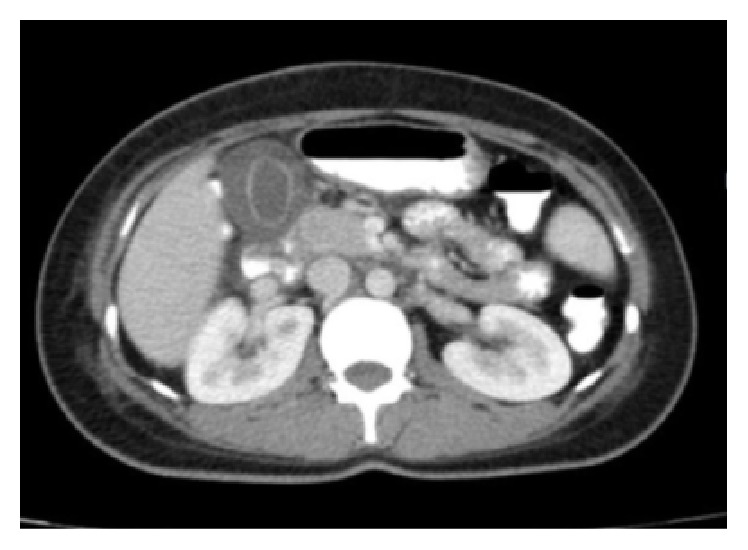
Abdominal CT scan representing an acalculous gallbladder with a thick wall.
